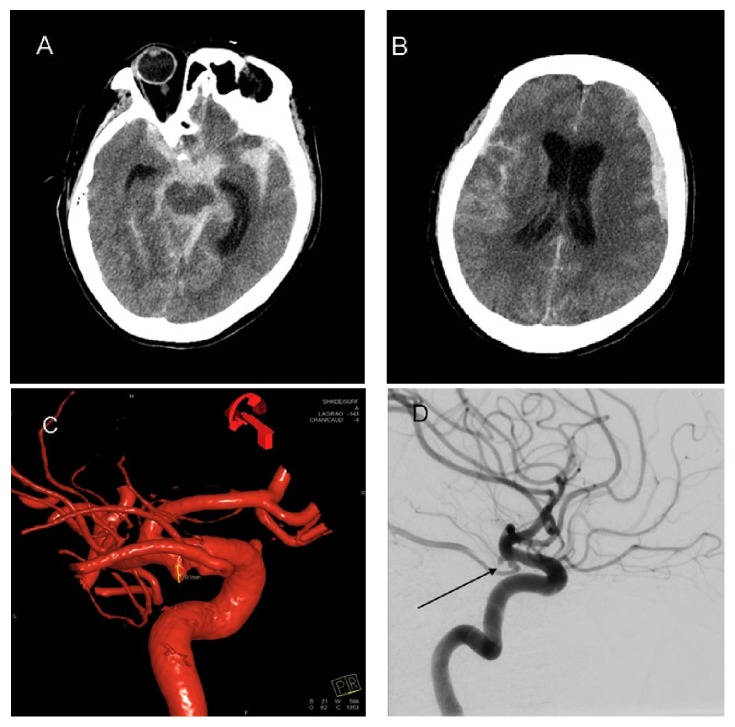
Admission CT and DSA. CT = computed tomography and DSA = digital subtraction angiogram. (A) Axial uninfused CT of the brain at the level of the mesencephalon displaying thick subarachnoid clot in the basal cisterns. (B) Axial uninfused CT of the brain at the level of the lateral ventricles displaying hydrocephalus, a small left acute subdural hematoma and diffuse SAH. (C) Lateral projection of left internal carotid artery injection via DSA displaying a small anterior choroidal artery aneurysm (black arrow). (D) Three-dimensional reconstruction of DSA displaying anterior choroidal artery aneurysm on the inferior portion of the supraclinoid internal carotid artery.
